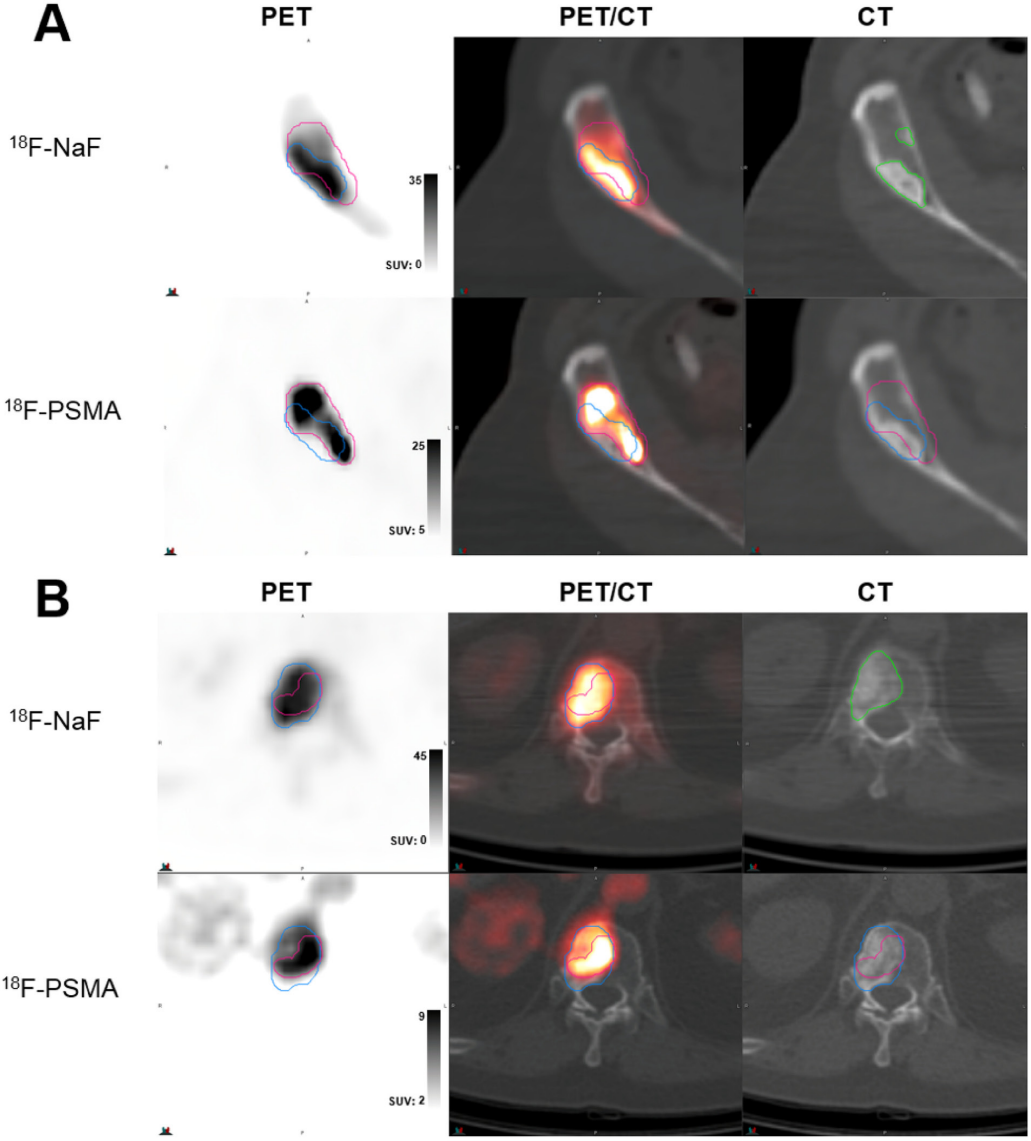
Examples of moderate-to-high volume concordance, with differential localization of highest uptake (A) Lesion in anterior iliac crest of castrate-resistant patient (serum PSA 93.3 ng/ml) demonstrating moderate 74.9% volumetric overlap between ^18^F-NaF uptake (top row, blue contour) and ^18^F-PSMA (DCFPyL) (bottom row, red contour) uptake, but mismatching patterns of high uptake within contours and areas of scelrosis on CT (green contour). (B) Lesion in T12 spine of castrate-resistant patient (serum PSA 388.1 ng/ml) demonstrating high 95.5% volumetric overlap between ^18^F-NaF uptake (top row, blue contour) and ^18^F-PSMA (DCFBC) (bottom row, red contour) uptake within visible CT lesion (green contour), but differential areas of highest uptake. In both (A) and (B), top row demonstrates PET uptake, PET/CT overlay, and low-dose CT from ^18^F-NaF scan and bottom row demonstrates PET uptake, PET/CT overlay, and low-dose CT from PSMA scans with registered contour overlay.
